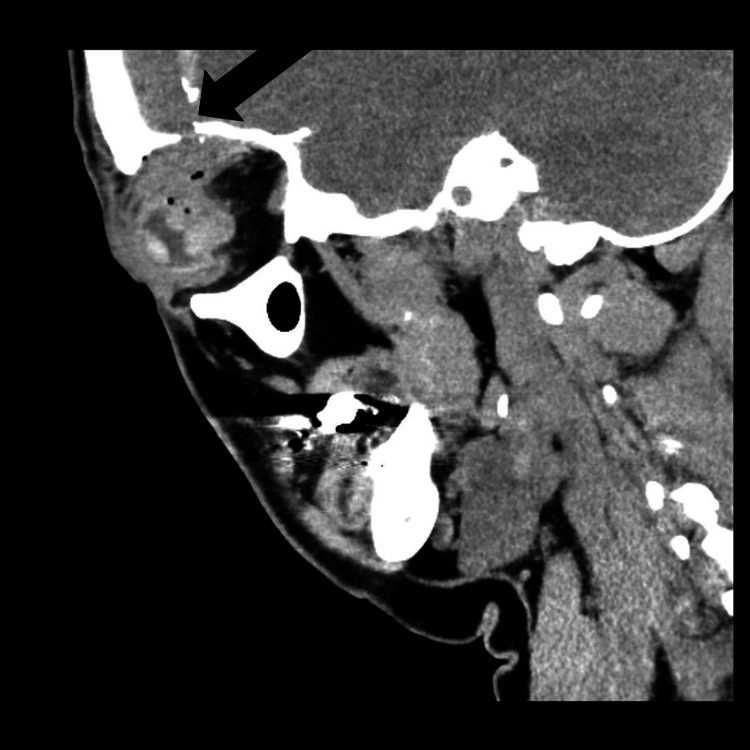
Orbital roof defect with bone fragments and hematoma on sagittal CT CT: computed tomography Sagittal CT images show a defect in the orbital roof, with displaced bone fragments and hematoma along the path of a foreign body (not visualized in this image), indicating traumatic penetration
